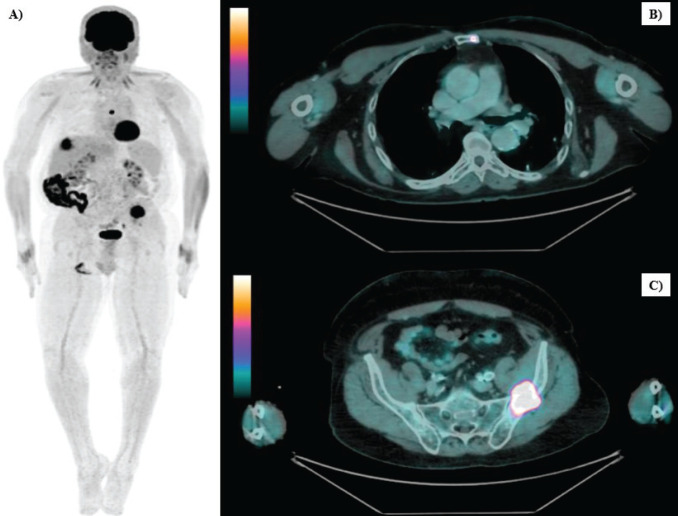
Whole-body FDG PET/CT images of secondary bone implants. (a): Whole-body maximum intensity projection (MIP) image showing abnormal FDG uptake in the left iliac bone wing, sternal region and a nodule in segment VII of the liver. (b): Sagittal view of a lytic bone lesion in the left side of the sternal body (SUVmax: 17.4). (c): Sagittal view of a metastatic implant in the left iliac bone (SUVmax: 18.5).
